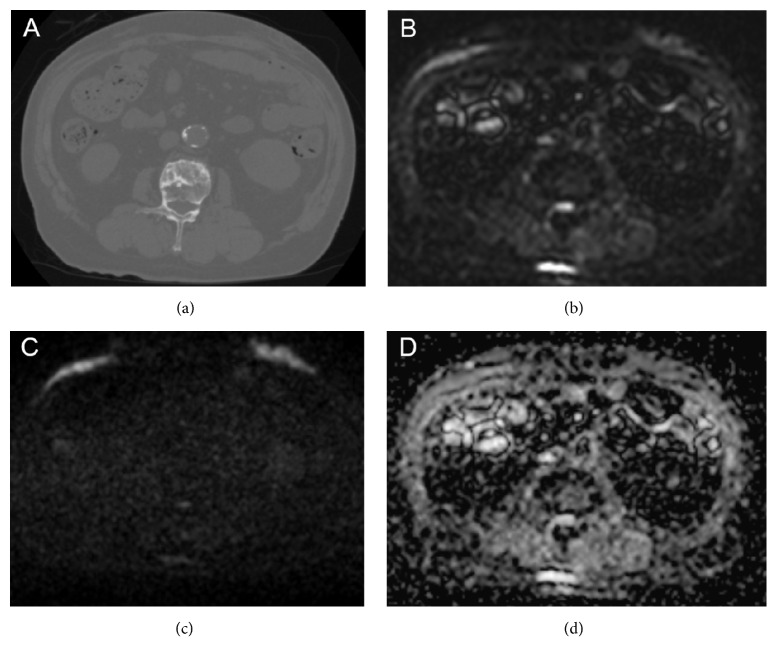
Imaging findings of MRI and CT in the second lumbar vertebra where CT-guided needle biopsy was performed. (a) CT revealed an osteoclastic lesion in the second vertebral body (885.4 HU). *b* = 0 (b) and *b* = 1200 (c) of DWI displayed no high signal intensity in the second lumbar vertebra. (d) The ADC map exhibited low signal intensity in the second lumbar vertebra (the ADC value was 0.498 × 10^−3 ^mm^2^/s). MRI, magnetic resonance imaging; CT, computed tomography; HU, Hounsfield unit; DWI, diffusion-weighted imaging; ADC, apparent diffusion coefficient.
